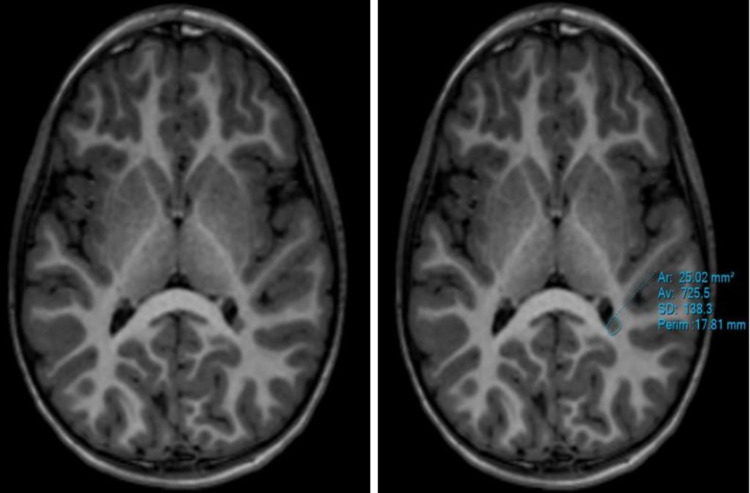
Axial T1-weighted MRI Measurement of the area of the subependymal heterotopia located at the level of the posterior horn of the left lateral ventricle was done.
